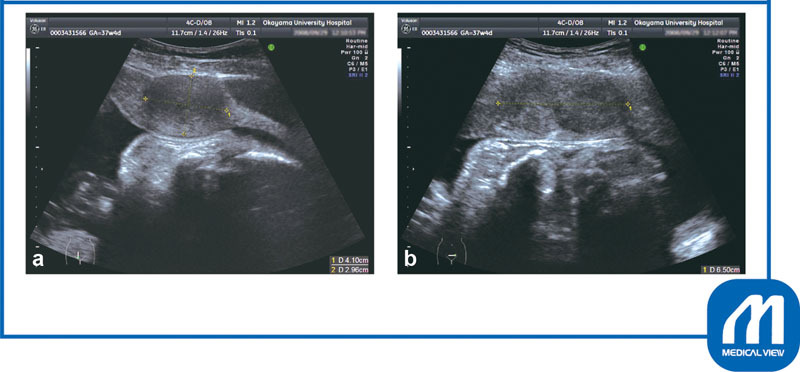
Case 2: ultrasonography findings. Two myomas (4-cm and 3-cm diameter) aligned in the anterior wall of the lower segment where the transverse incision is usually made.
